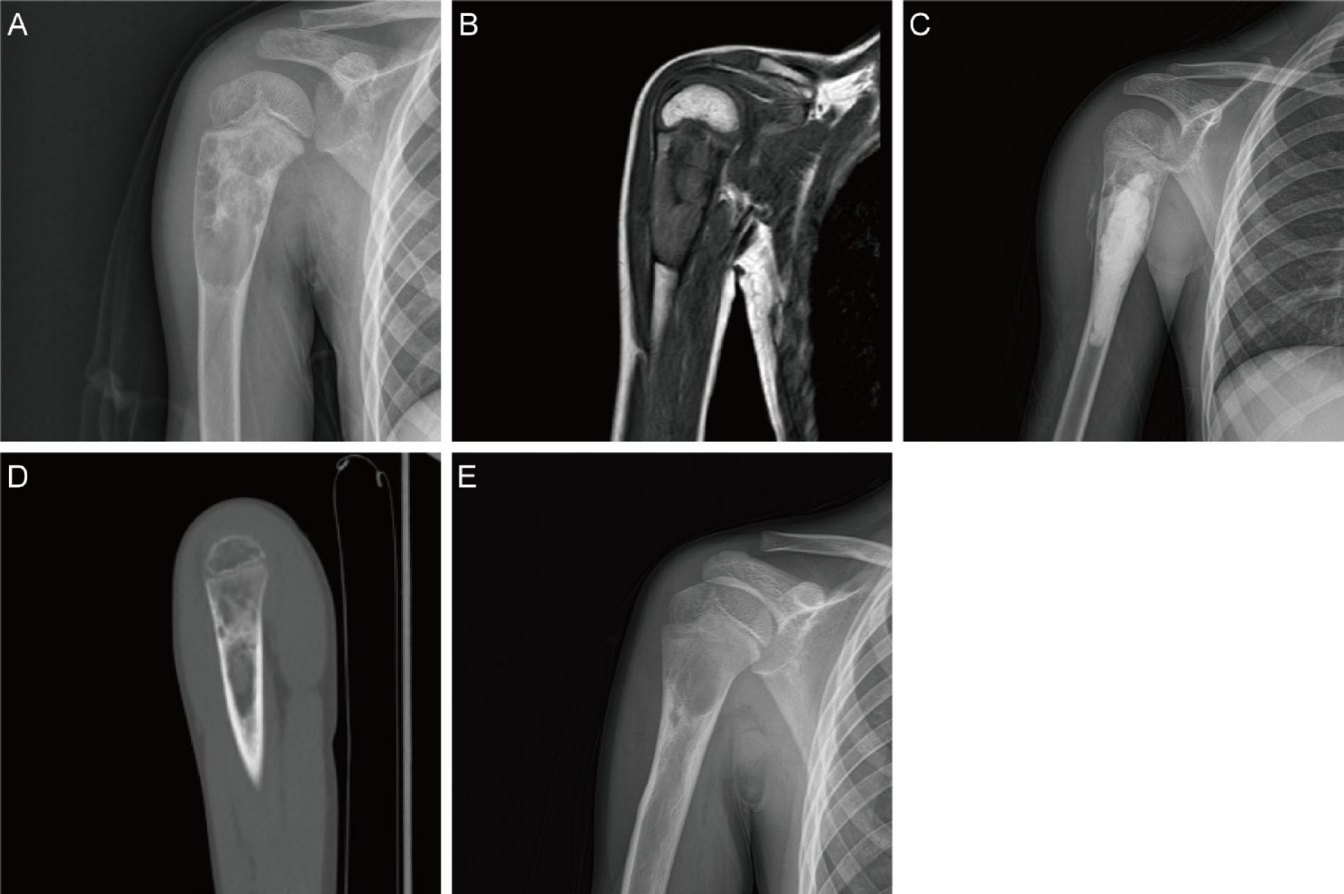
A, B Preoperative X-ray and MRI images of the humerus UBC in a 9-year-old boy. C Postoperative X-ray images on the day after surgery. D Postoperative CT images at 12 months of follow-up. E Postoperative X-ray images at 30 months of follow-up showed partial healing
